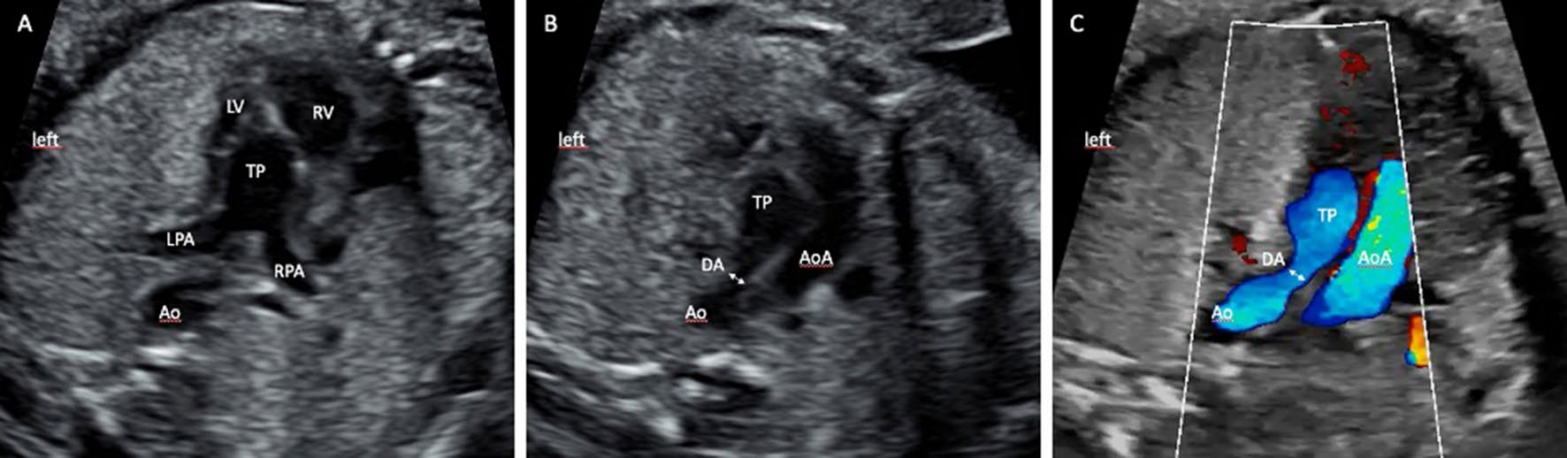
DA in the 3-vessel view in B-mode and Color Doppler. A ventriculo-arterial discordance with the pulmonary trunk arising from the left ventricle, B 3-vessel view with restrictive arterial duct, the arrow shows the size of the arterial duct, C 3-vessel view in Color Doppler, showing an antegrade perfusion of the restrictive arterial duct. (*LV* left ventricle, *RV* right ventricle, *TP* main pulmonary trunk, *LPA* left pulmonary artery, *RPA* right pulmonary artery, *Ao* descending aorta, *AoA* aortic arch)
